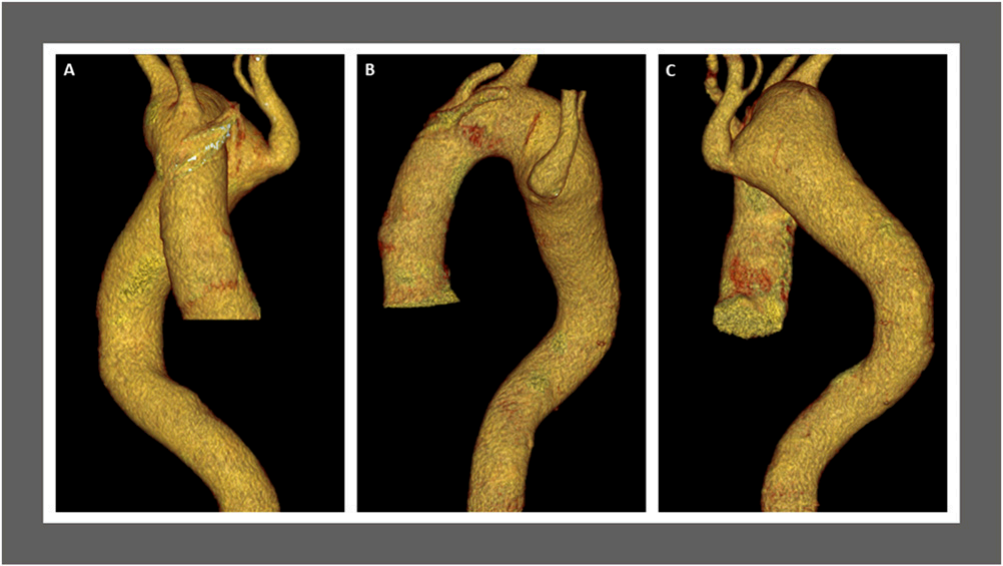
Preoperative computed tomography angiogram findings. Anterior view (A), left lateral view (B), and posterior view (B) of three-dimensional reconstruction with volume rendering technique demonstrate the arch vessels, ascending and descending aorta with aneurysmal dilatation due to diverticulum of Kommerell adjacent to an aberrant left subclavian artery at the origin of the aberrant left subclavian artery. The branches from the anterior aspect of the right-sided aortic arch are the left internal carotid artery, the right internal carotid artery, and the right subclavian artery. The aortic arch measures 3.7 cm in diameter. The distal aortic arch measures 5.2 cm in maximum transverse diameter. The diverticulum of Kommerell measuring maximum angle corrected diameter of 58 × 41-mm at the origin of the aberrant left subclavian artery.
